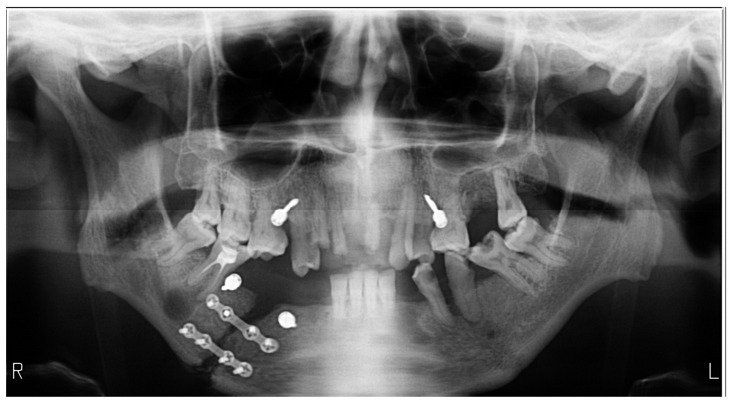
Orthopantomography of a case with a double fracture of the mandible, left subcondylar, and lateral on the right side, the later surgically reduced and fixed, exemplifies the head in flexion error, with poor visibility in the menton region.
